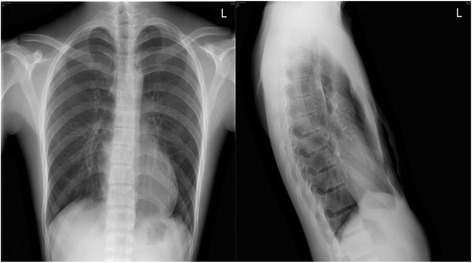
Chest roentgenologic image showed compression of the heart by pectus excavatum
